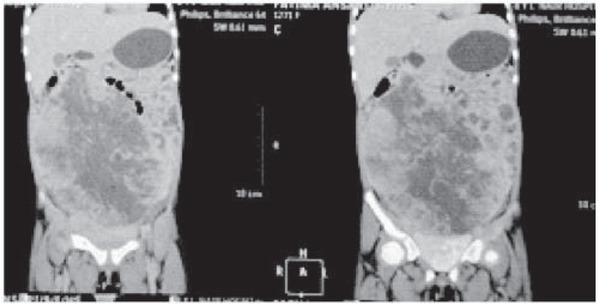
CT scan (Saggital section) showing large solid cystic adnexal/ovarian mass with septations and peritoneal deposits.
